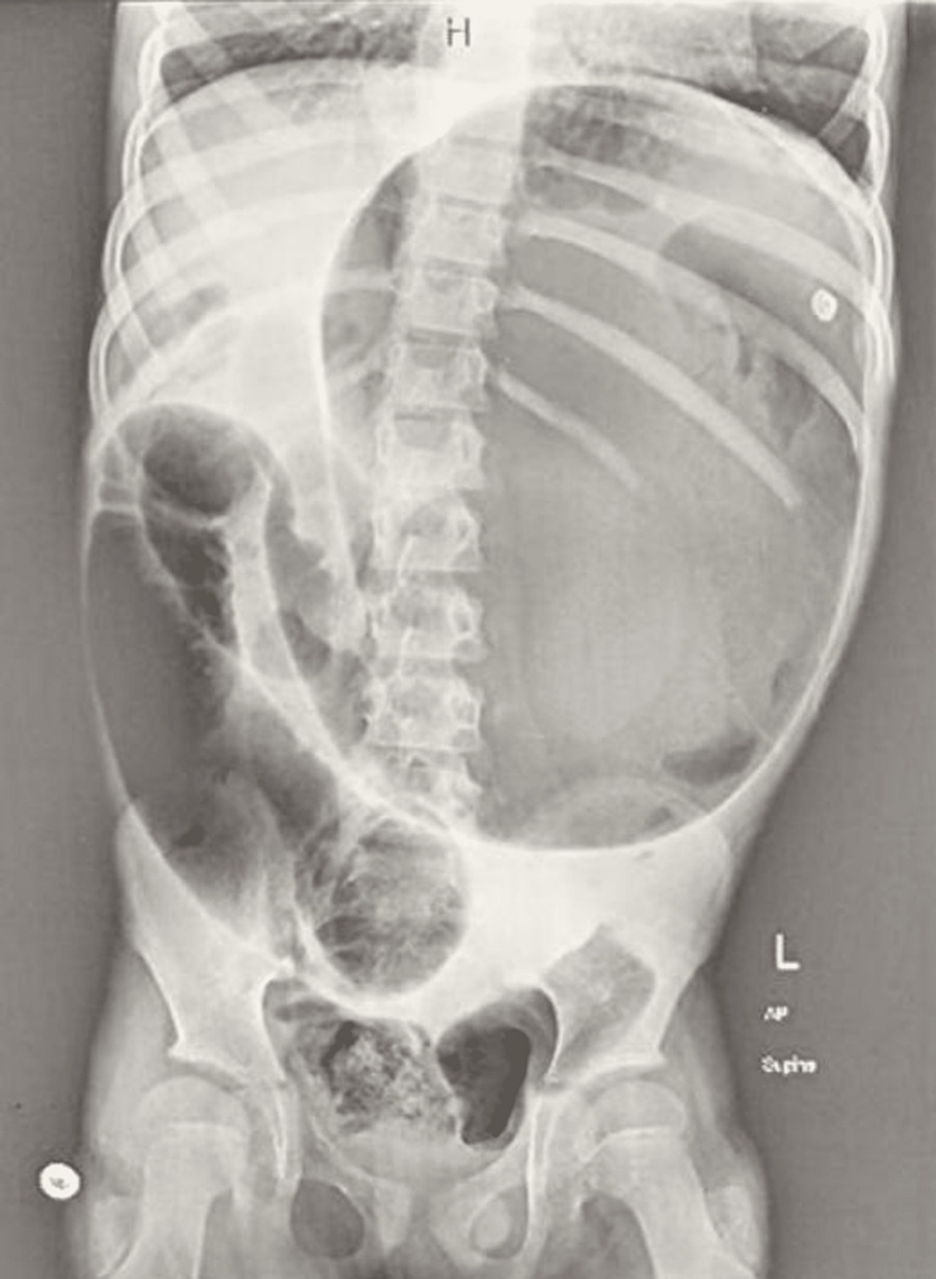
Plain radiograph of the abdomen Significant distension of the stomach and adjacent duodenum is seen. A small amount of air and fecal material can also be appreciated in the sigmoid colon
